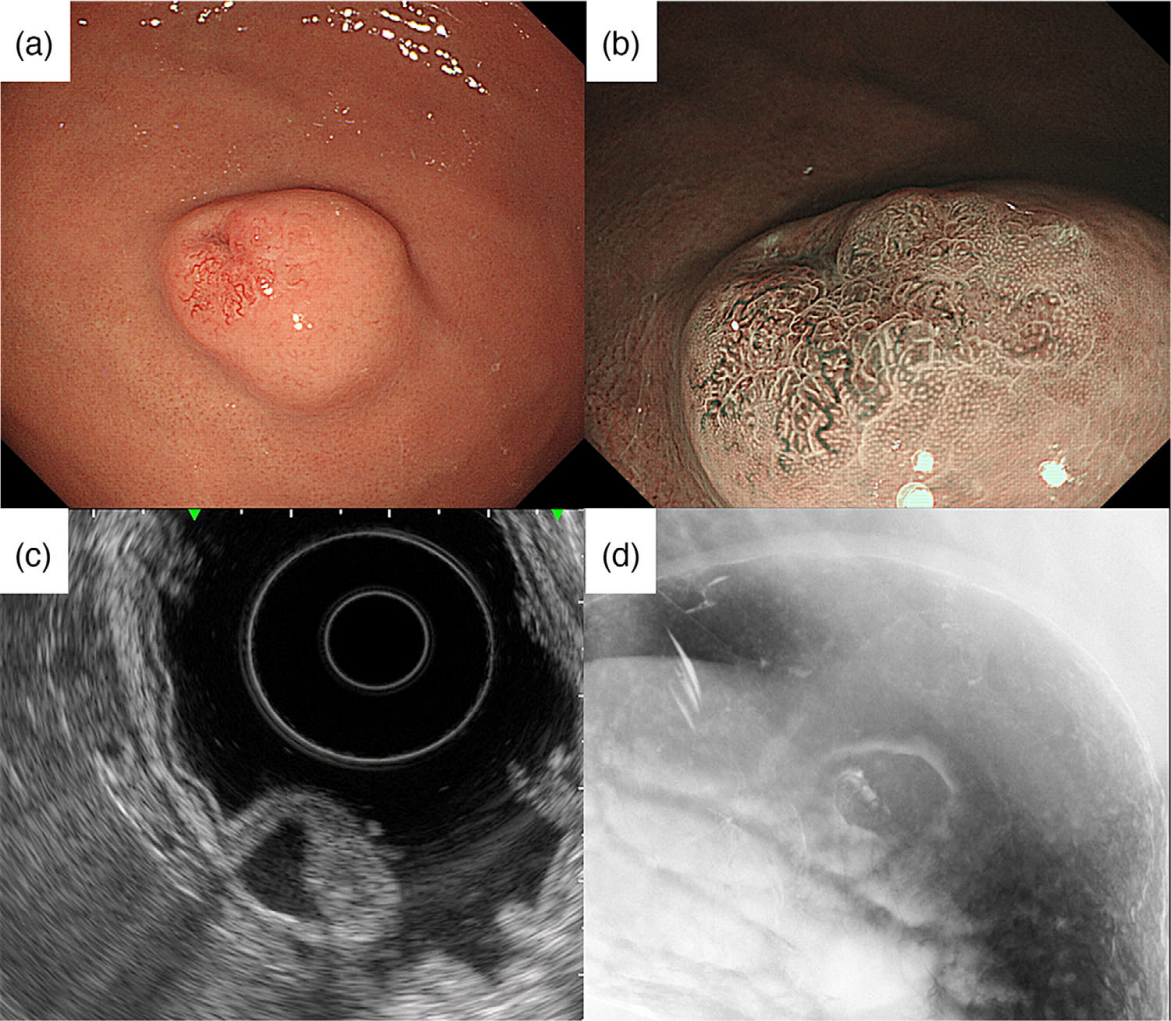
(a) EGD revealed a gastric SMT of 20 mm in diameter in the greater curvature of the upper gastric body with a depression and dilated vessels. (b) Magnifying endoscopy with narrow‐band imaging detected a redness area around the depression having a heterogeneous arrangement of irregular villi and pit pattern without a clear demarcation line. (c) Endoscopic ultrasonography disclosed a heterogeneous tumor with cystic areas located in the third layer. (d) Upper gastrointestinal X‐ray series showed a SMT in the upper gastric body with an irregular depression on its surface
